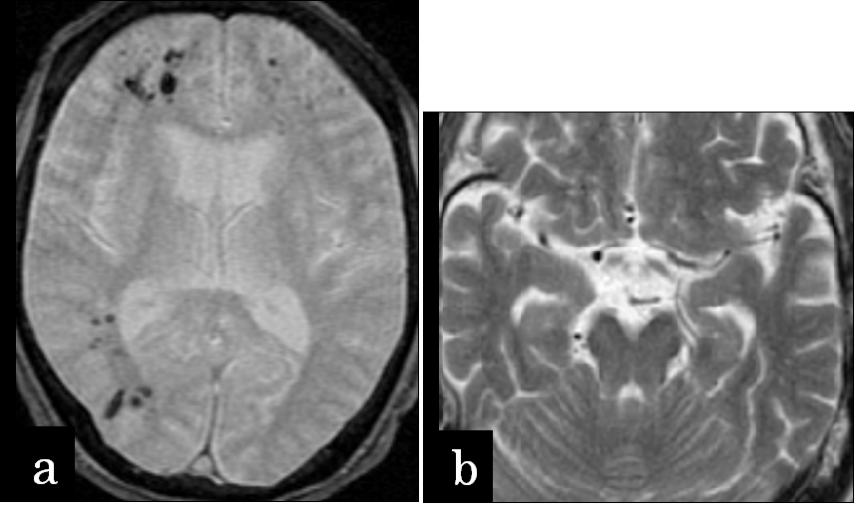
Cranial MRI (T2). Small collections of gas were revealed within the right hemisphere and frontal lobe (a) and internal carotid artery siphon (b).
